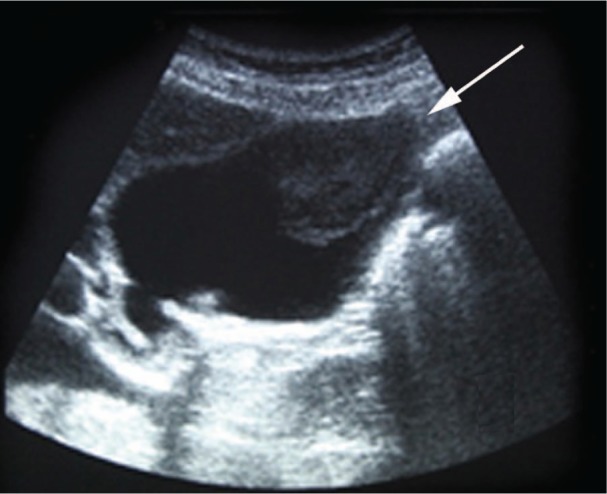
Case 2. Ultrasonography image with immobile mass in the fundus (arrow).
